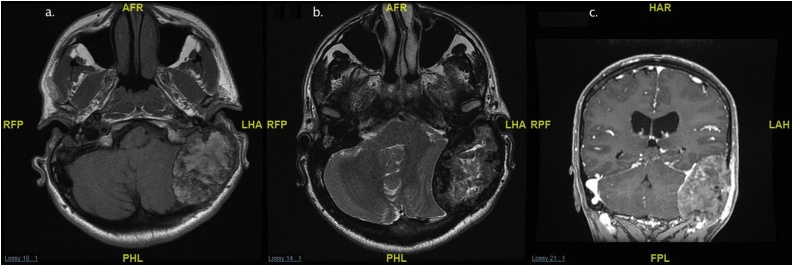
a. T1 without IV contrast. b. T2 weight image. c. Coronal T1 weighted image with IV contrast. Magnetic resonance imaging (MRI) of the temporal bone identified a large, expansile, heterogenous mass in the left tympanomastoid region with involvement of the mastoid air cells, and occipital bone and a mass effect on the cerebellum and left posterior temporal and occipital lobes. The mass showed very tiny foci of enhancement (c) and had intrinsic high-signal intensity on T1 and T2-weighted imaging. A similarly limited but smaller mass was found on the right side.
